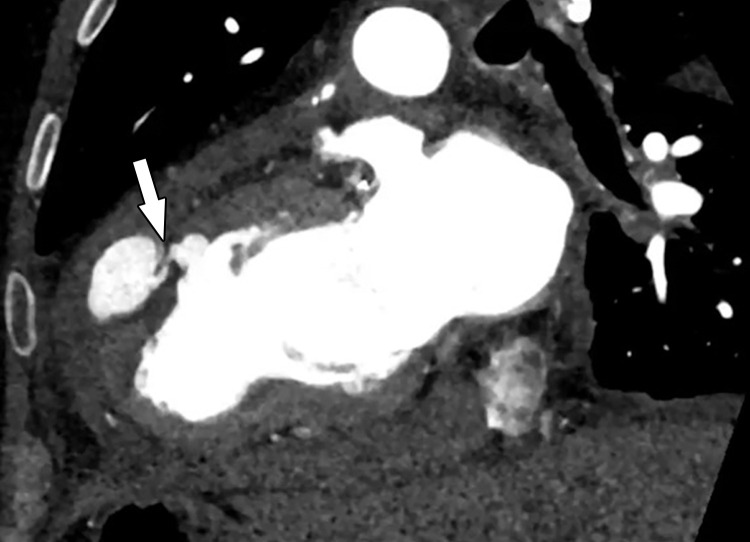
Pseudoaneurysm in a 61-year-old man with worsening chest pain 2 weeks after coronary artery bypass surgery who was referred for CT angiography for further evaluation. CT angiographic image shows an outpouching of contrast material with a narrow neck (arrow) arising from the LV, compatible with a pseudoaneurysm.
